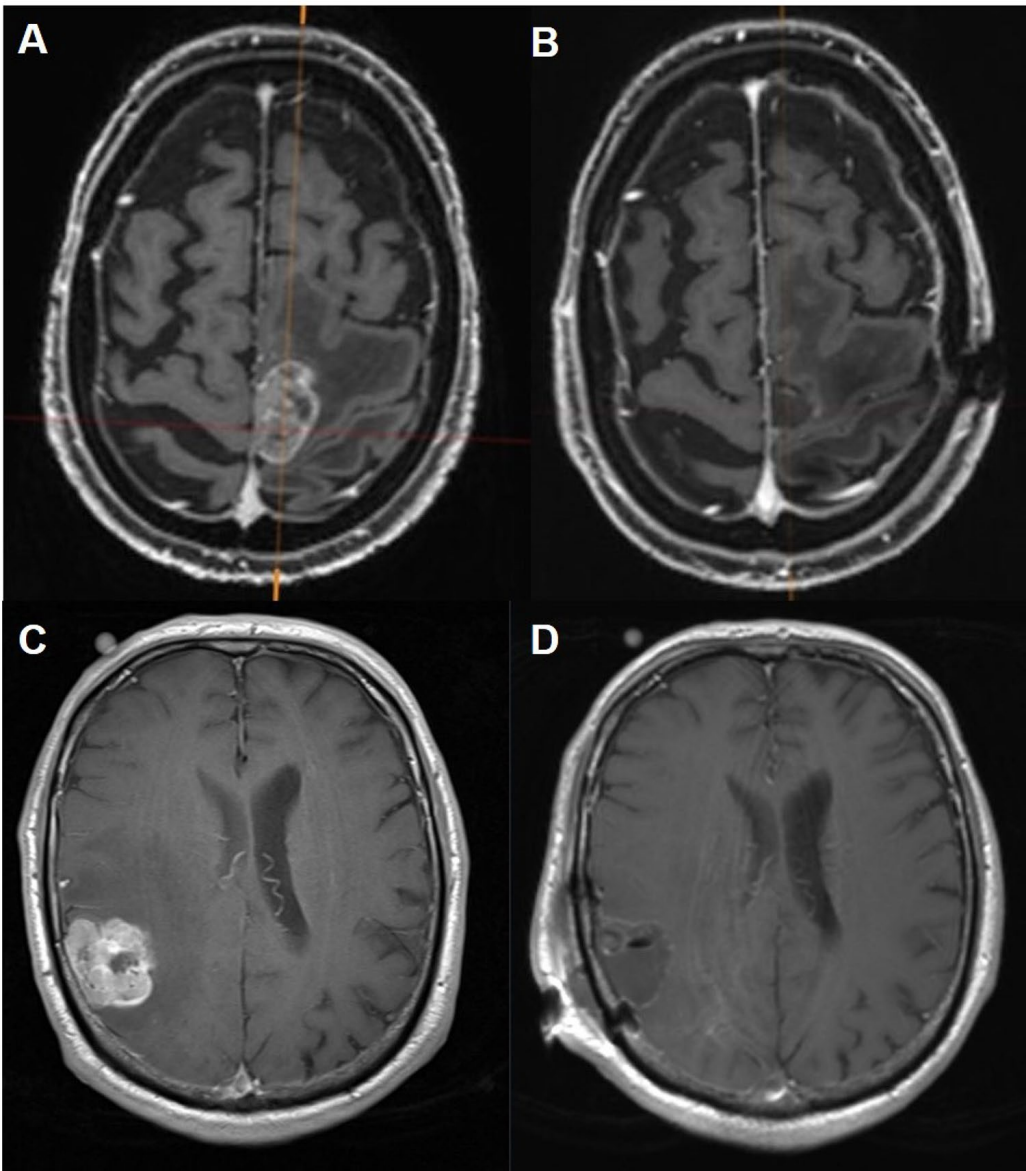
Resection of eloquent and non-eloquent metastases. Axial T1-weighted MR images with contrast are displayed with eloquent (A and B) and non-eloquent (C and D) metastasis. Eloquent metastases (A) were resected without a safety margin, resulting in a proportionally smaller postoperative resection cavity (B). In contrast, non-eloquent metastases (C) were resected with an additional safety margin, resulting in a larger resection cavity (D)
